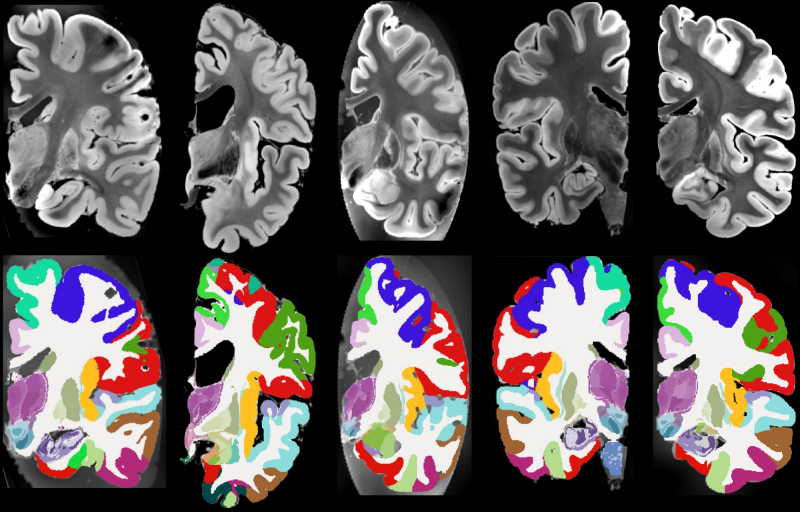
Five example subjects from the ex-vivo MRI data set. Coronal view of the MRI data (top) and the corresponding automated segmentation using NextBrain (bottom).
